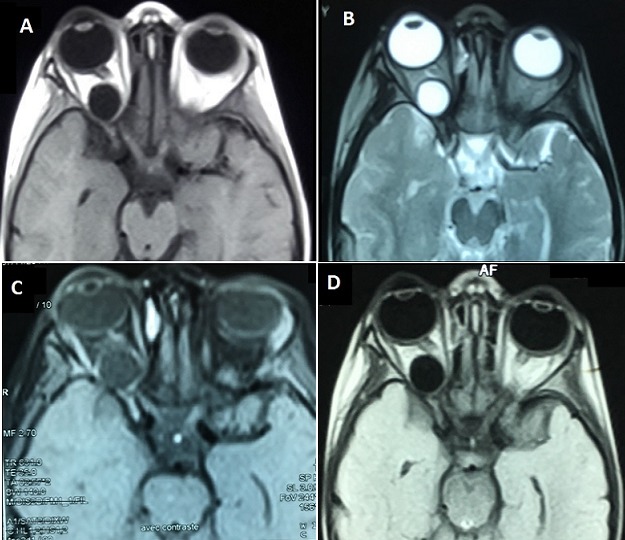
Brain MRI Axial sequences: T1 (A), T2 (B), T1 with contrast injection (C) and Flair Sequence (D) objectifying a rounded lesion that appears hypointense T1, hyperintense T2, well limited, thin walled Which takes slightly the contrast product (C), on the Flair sequence (D) it appears hypointense testifying to the liquid content of the lesion, which laterally compresses the optic nerve
